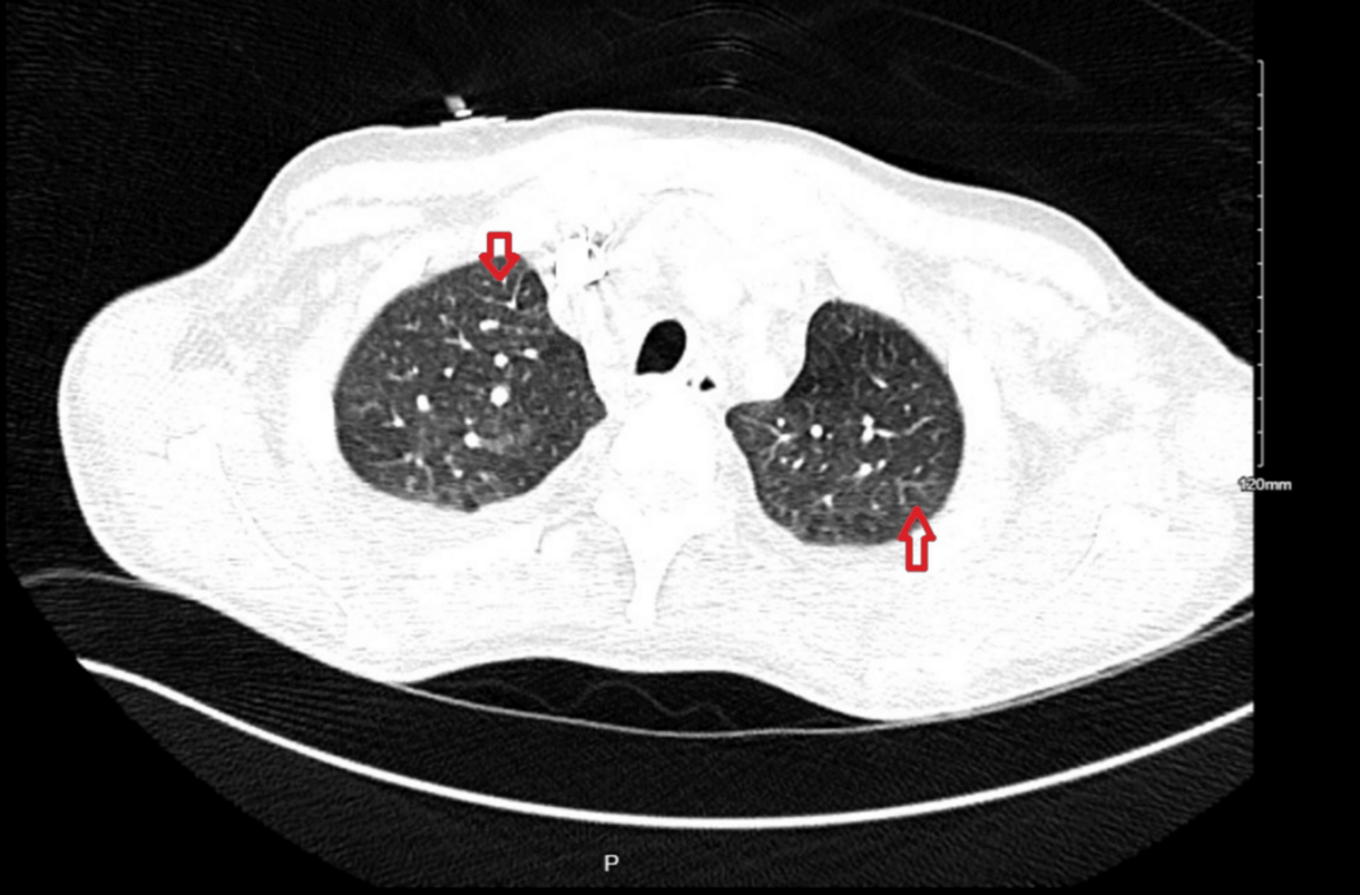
CT angio chest (axial view) Red arrows: tree-in-bud appearance in bilateral upper lung lobes CT, Computerized Tomography
